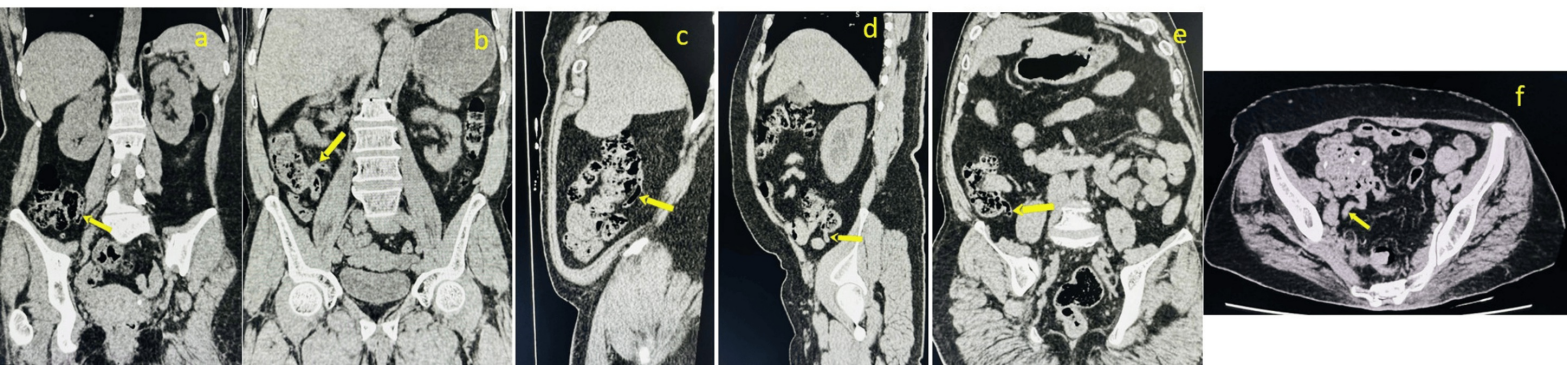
Anatomical positions of the appendix in the NCCT (a) Retroileal position; (b) Preileal position; (c) Retrocaecal position; (d) Subcaecal position; (e) Preileal position; (f) Pelvic position NCCT: non-contrast computed tomography
